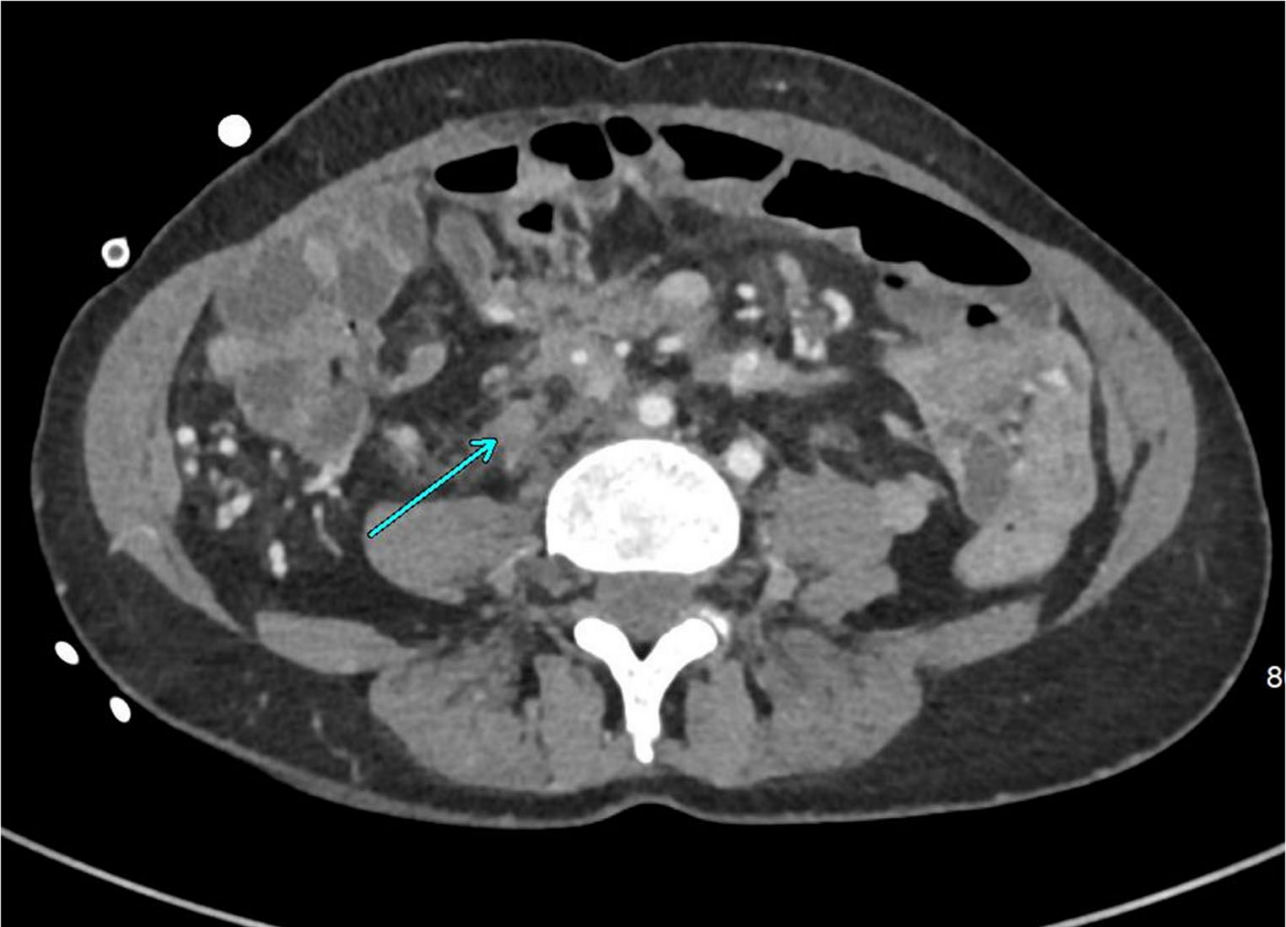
CT axial image, arrow indicates: right ureter encased with a desmoid tumour
